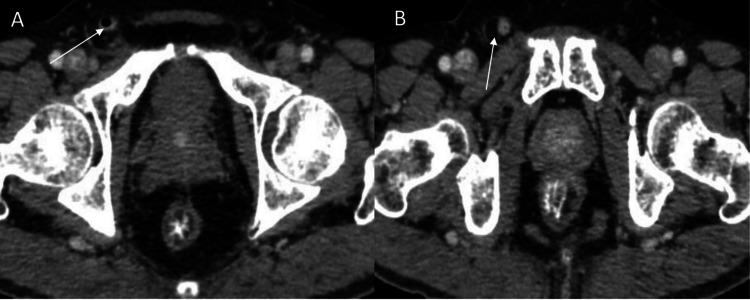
Axial CECT images showing the appendix arising from the cecum entering into the right inguinal canal (A and B, white arrow) CECT: contrast-enhanced computed tomography
